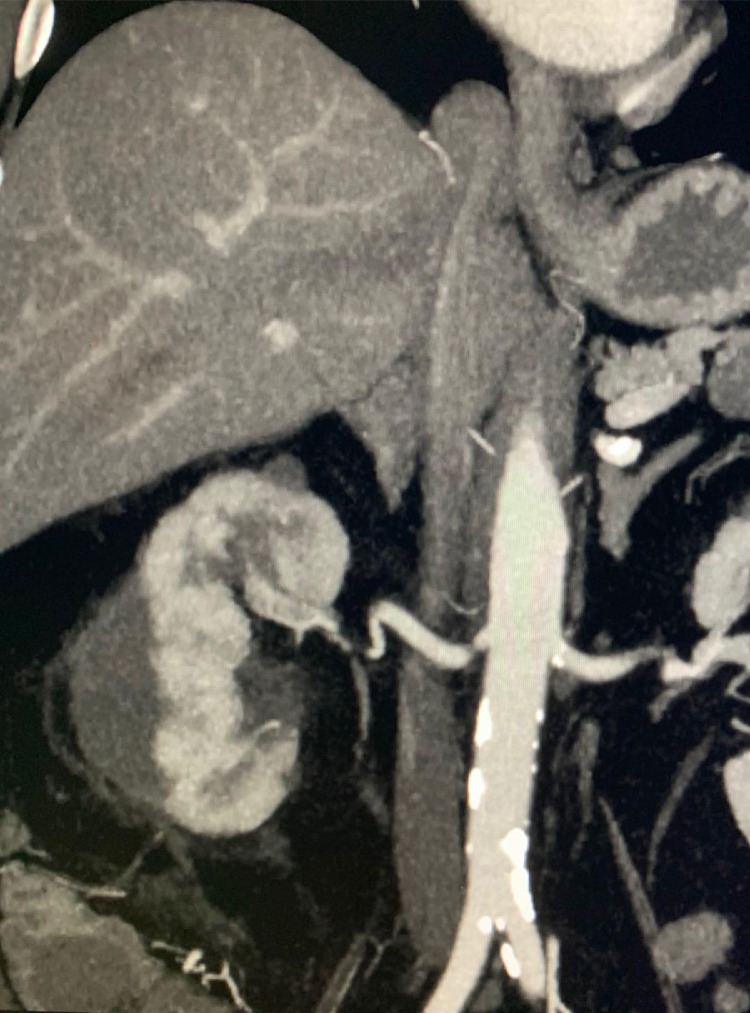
Coronal CT with contrast at 6-week follow-up which demonstrates a decrease in size of the subcapsular hematoma with interval resolution of the pseudoaneurysms.
